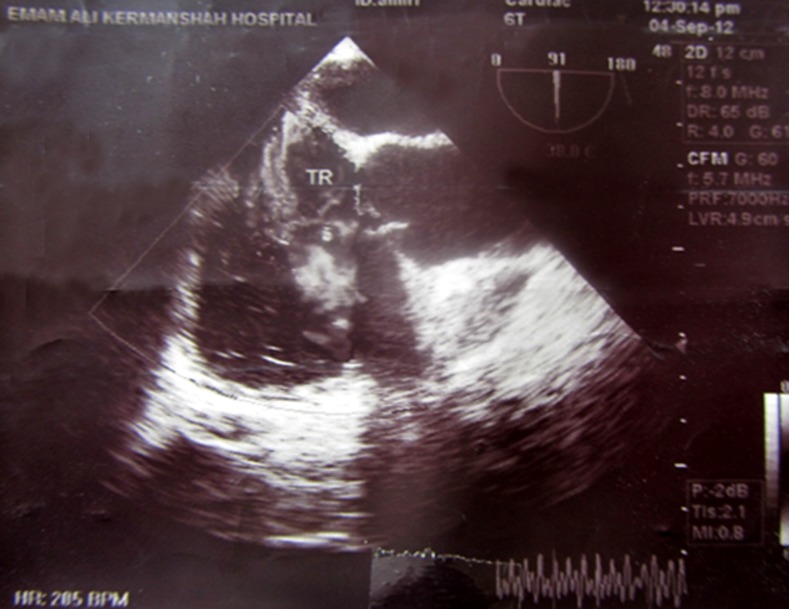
Transesophageal Doppler study revealing severe tricuspid regurgitation
